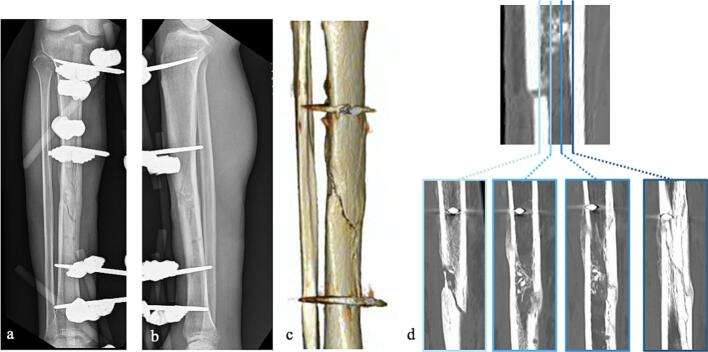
Radiological findings 5 months after surgery. a: Anteroposterior plain radiograph. b: Lateral plain radiograph. c: Three-dimensional computed tomography reconstruction. d: Axial computed tomography image.
